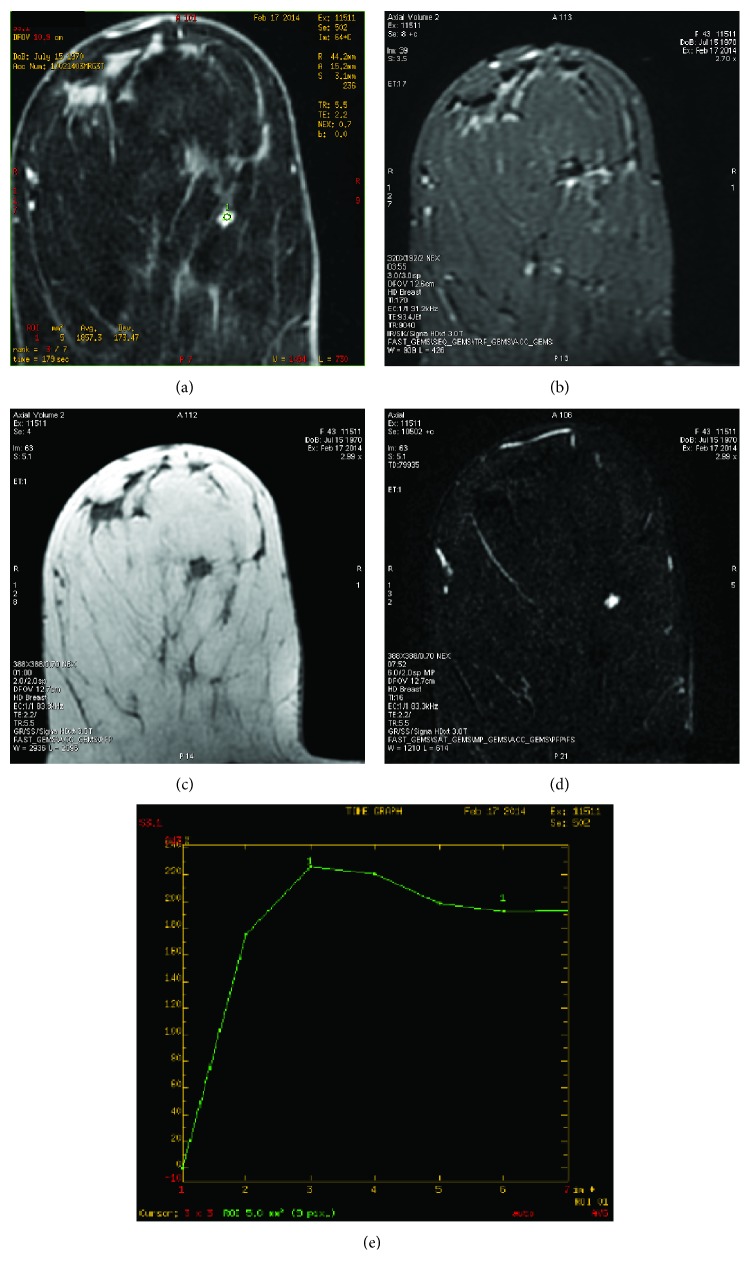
Contrast-enhanced MRI imaging of a cavernous hemangioma of the breast mimicking an invasive lesion. (a) Τ1W fat sat postcontrast image showing a small enhancing lesion measuring 5 mm, with irregular margins in the lower inner quadrant of the right breast. (b) STIR image showing the mass with low signal and central high signal. (c) Precontrast T1W non fat suppressed image showing an intermediate signal in the lesion. (d) Postcontrast T1W subtraction image: a small enhancing mass is seen. (e) A type III curve with rapid enhancement and delayed washout is observed.
